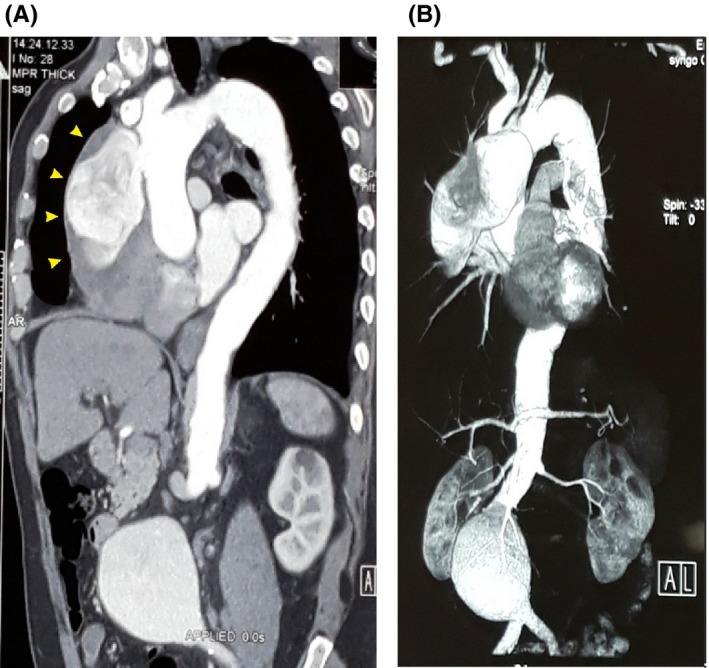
A, Contrast‐enhanced CT scanning and B, CT angiography of the chest and abdomen in case 1. A large saccular aneurysm of the ascending aorta (yellow arrowheads) and a fusiform aneurysm of the abdominal aorta are shown
